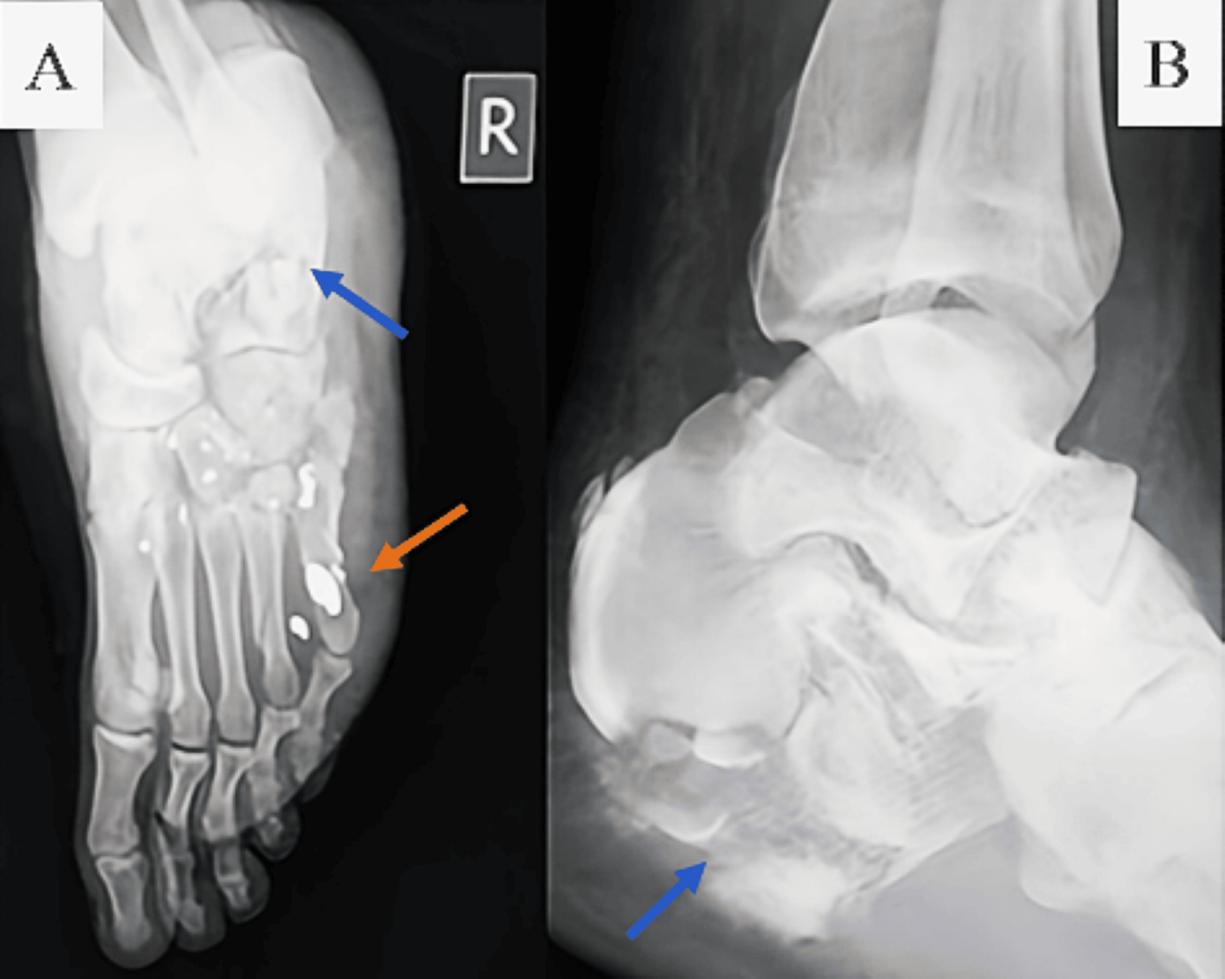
Standard radiographs of the right foot and ankle demonstrating a closed blast injury with comminuted calcaneal fracture. (A) The anteroposterior view shows extensive calcaneal fragmentation (blue arrow), with multiple radiodense foreign bodies consistent with metallic fragments from a blasting cap (orange arrow). (B) The lateral view confirms the comminuted nature of the calcaneal fracture.
